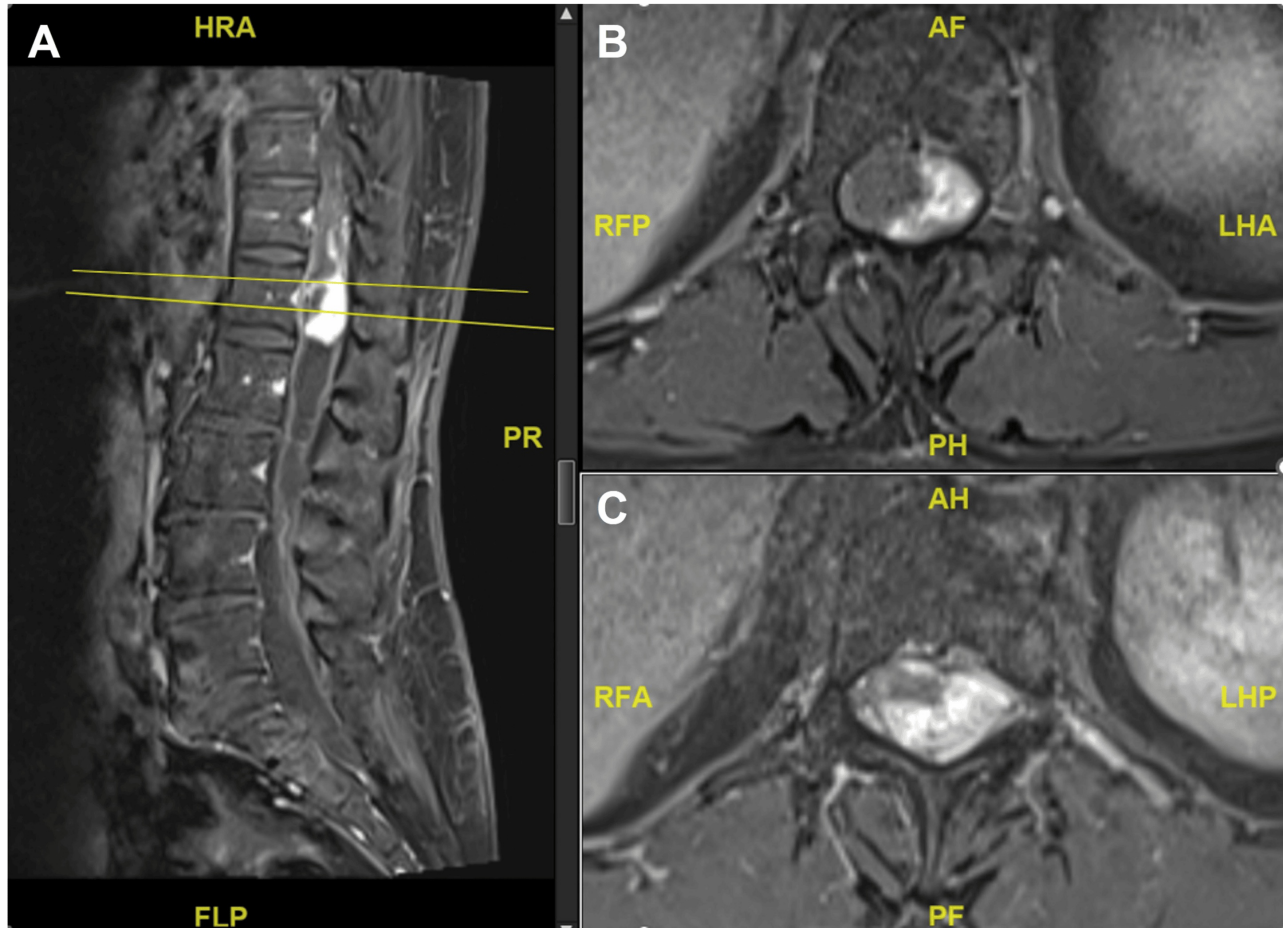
T1-weighted imaging with contrast MRI of the lumbar spine revealed a lobulated heterogeneously enhancing solid-cystic lesion within the spinal canal at the T11-12 levels.
